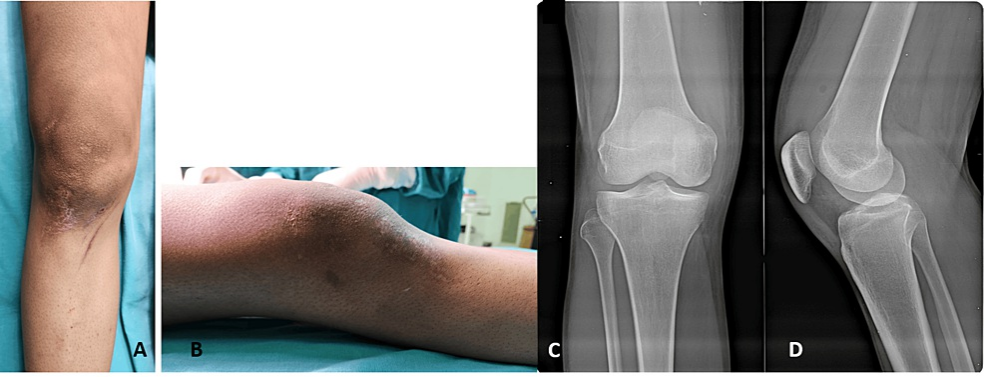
Clinical pictures and X-rays at the time of presentation. Clinical pictures (A & B) showing moderate effusion of the right knee. Antero-posterior view (C) and lateral view (D) of radiograph showing no obvious bony injury.
